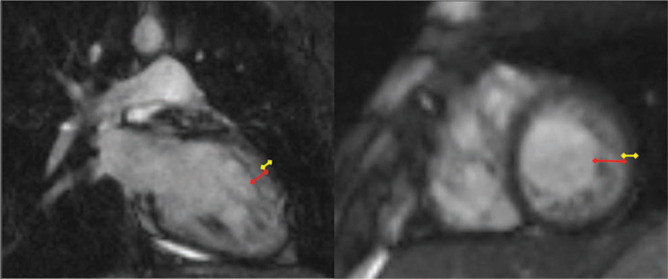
Cardiac MRI images of a patient with IVNC, demonstrating NC:C ratio of 3.7:1 (diagnostic 2.3:1).
